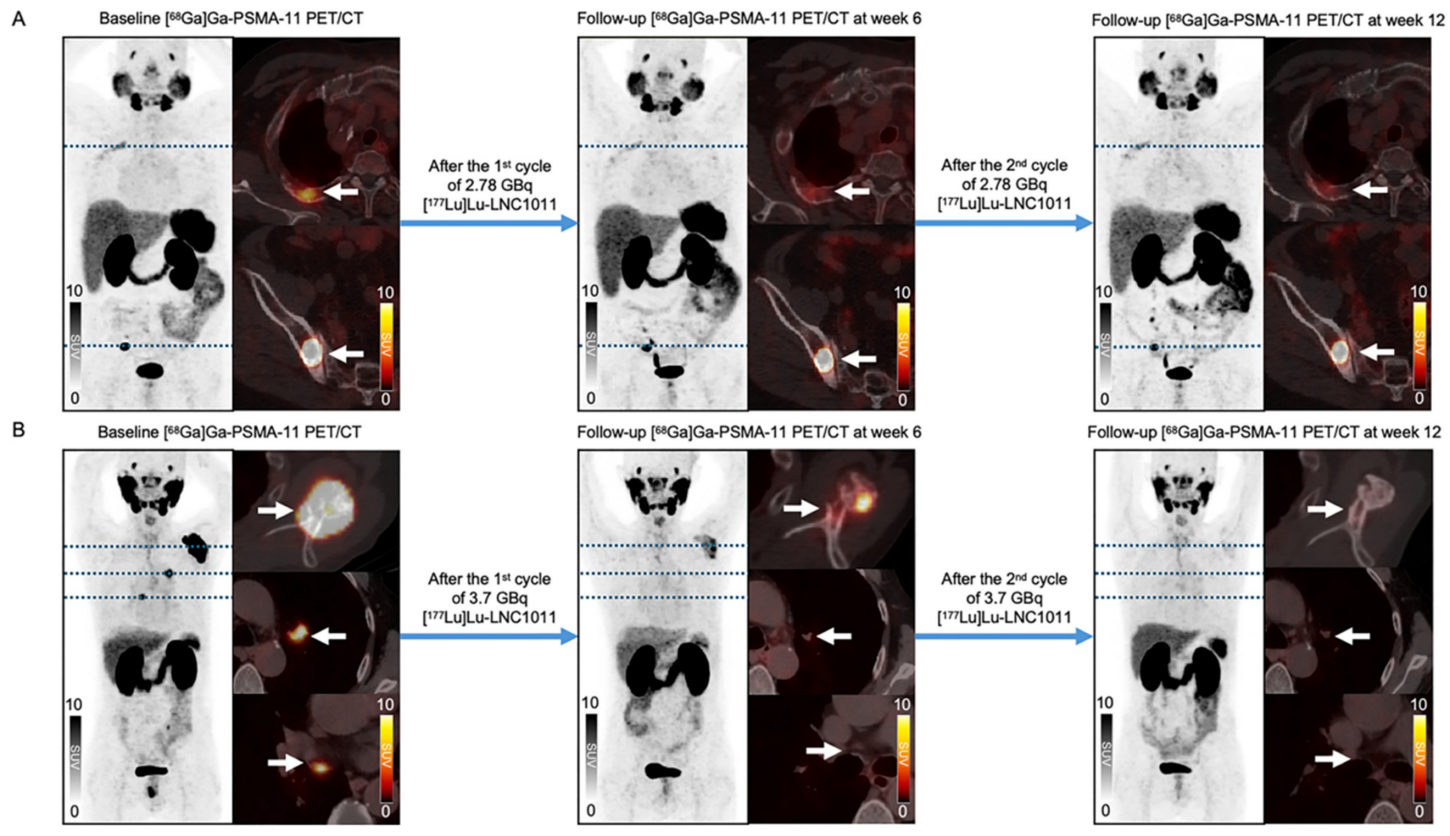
Representative patients' treatment responses: (A) In the 2.78 GBq cohort, a 76-year-old male patient (No. 6) exhibited a bone metastasis lesion in the right iliac. After 2 treatment cycles, the lesion's SUVmax decreased from 62.18 to 37.19 and further to 34.03, accompanied by a decline in PSA levels (from 6.66 to 5.86 to 2.85 ng/mL) and PSMA-VOL reduction (from 7.5 to 6.4 to 5.04 cm³). (B) In the 3.70 GBq dosing cohort, a significant reduction in uptake was observed in the left scapula of a 65-year-old male patient (No. 7), with the SUVmax decreasing from 79.26 to 10.59 to 2.25. The patient's PSA levels changed from 21.1 to 0.52 to 0.013 ng/mL, and PSMA-VOL decreased from 16.18 to 10.98 to 2.31 cm³.
